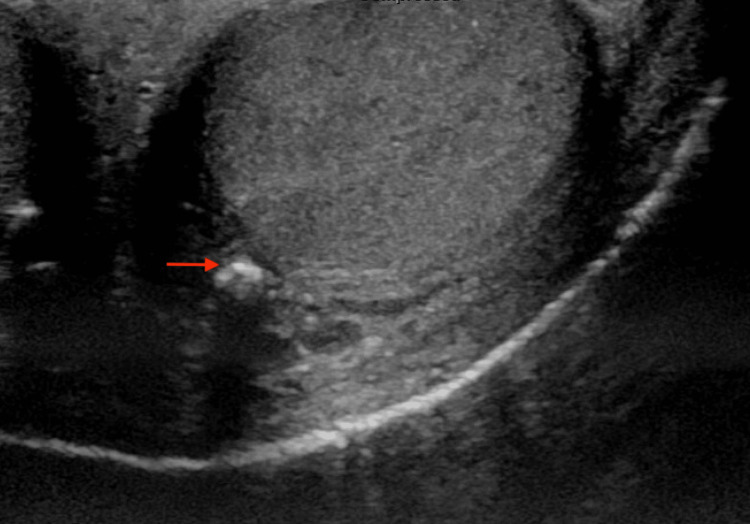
Scrotal ultrasound Arrow depicts calcifications identified on ultrasound
